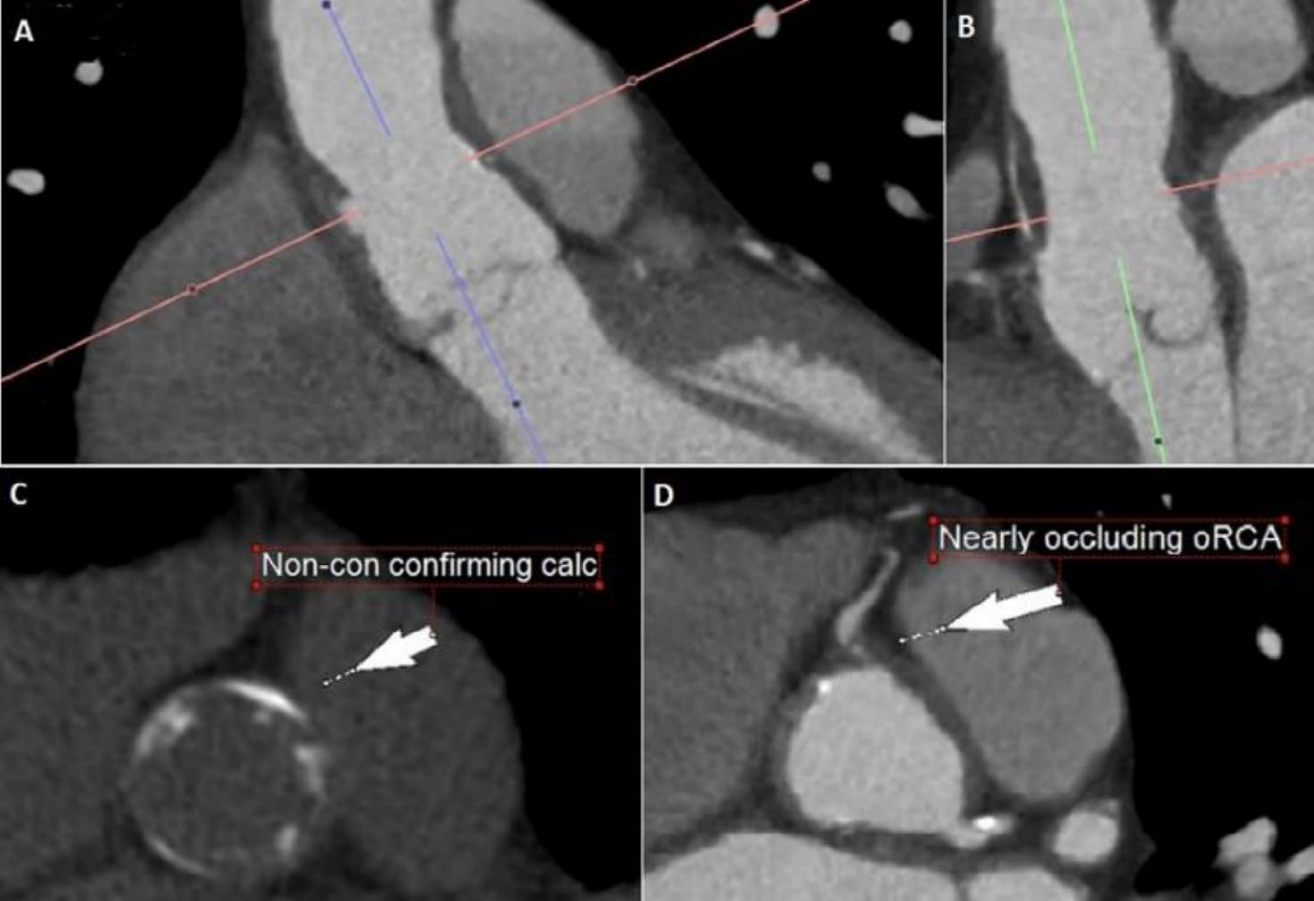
Computed tomography coronary angiography revealing two separate small penetrating aortic ulcerations in the aortic root (A, B) and associated severe burden of atherosclerosis in the aortic wall (C), with subsequent compression and near‐occlusion of the ostium of the right coronary artery (oRCA) (D).
